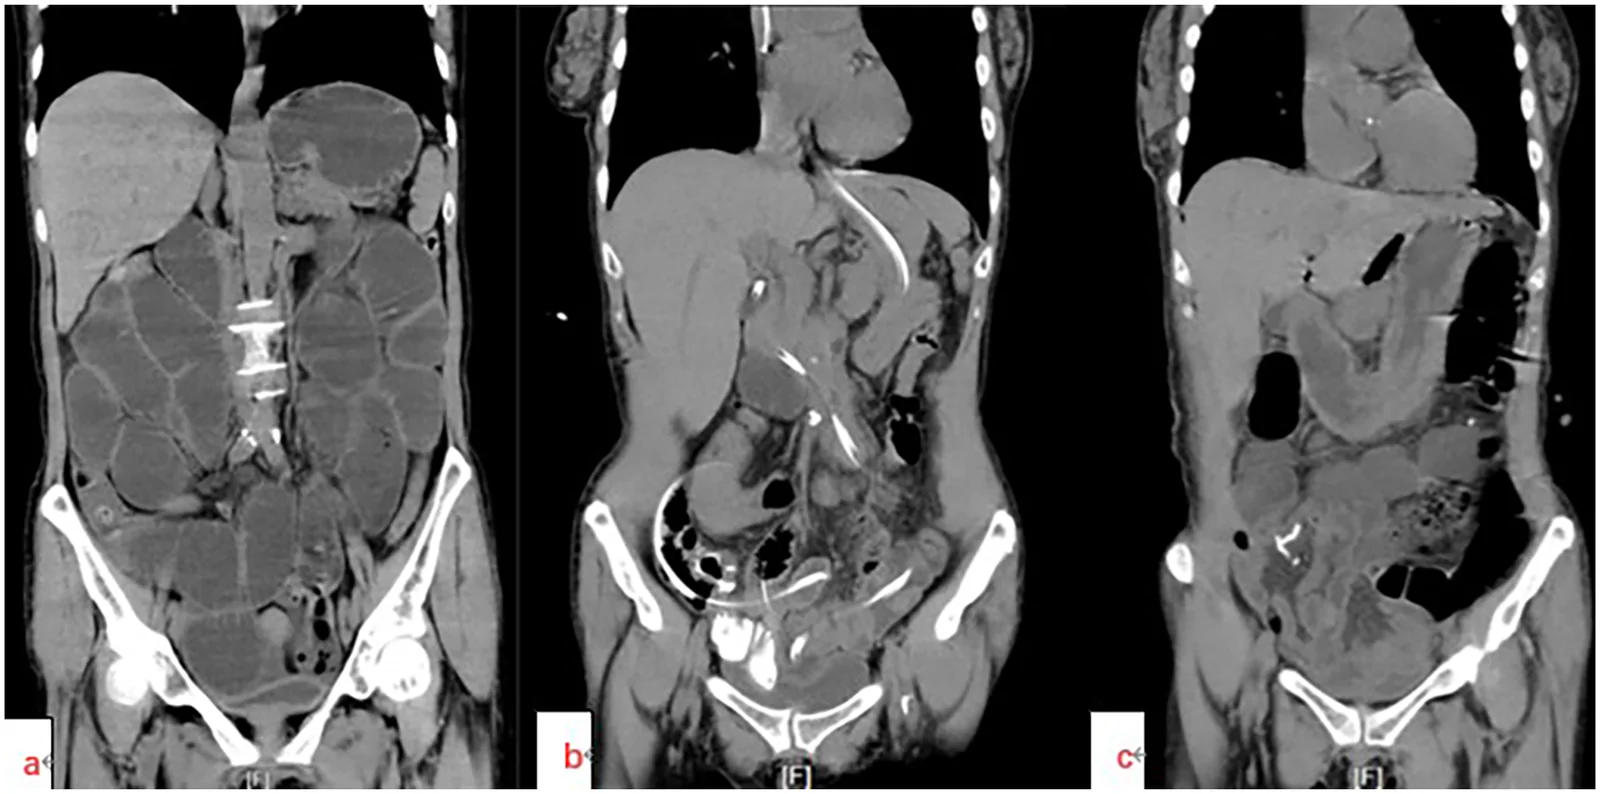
Contrast-enhanced abdominal CT scans. (a) At the first episode of obstruction (November 2024): dilated small bowel loops with a transition point. (b) Obstruction recurrence after tube removal (December 2024): persistent small bowel obstruction. (c) Resolution of obstruction after surgical resection (December 2024).
